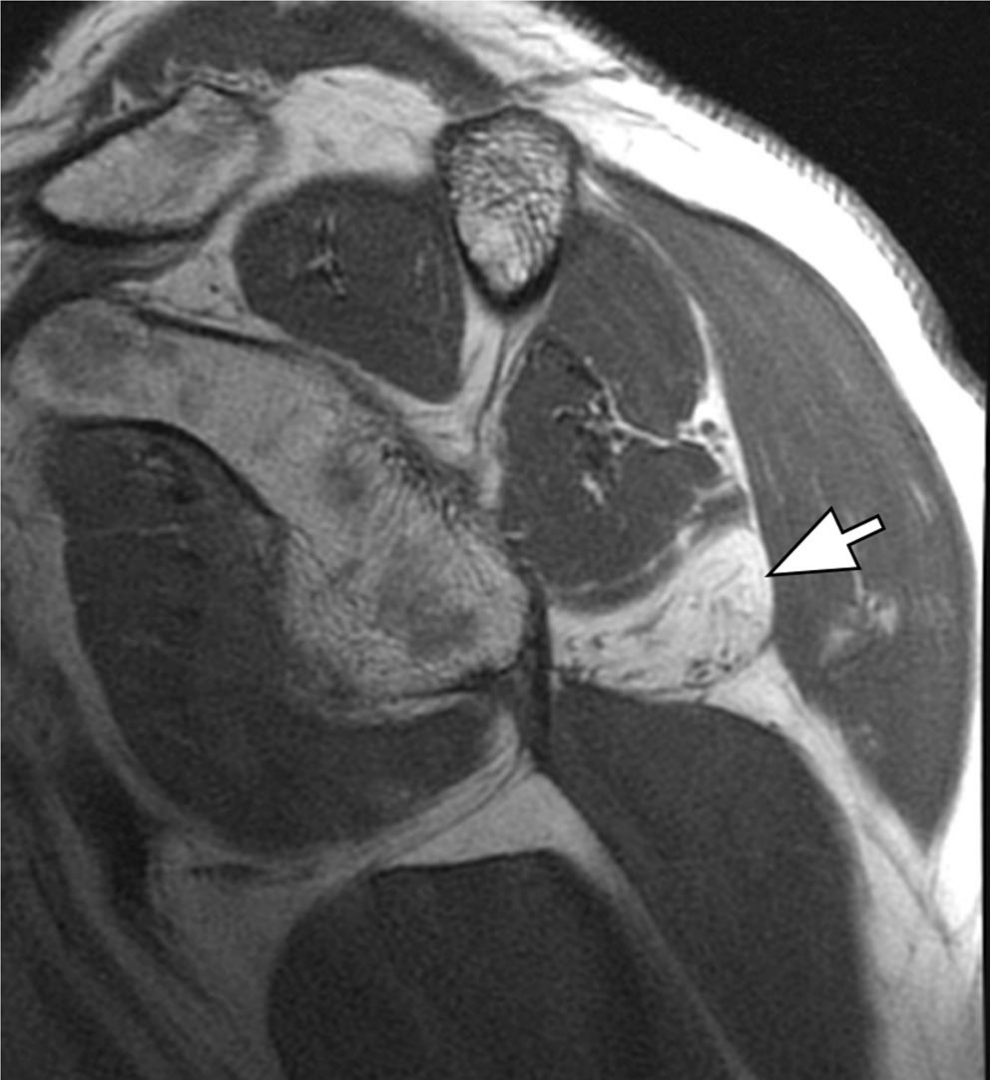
Quadrilateral space syndrome. Sagittal T1-weighted image of the shoulder. Complete fatty atrophy of the teres minor muscle (arrow)
